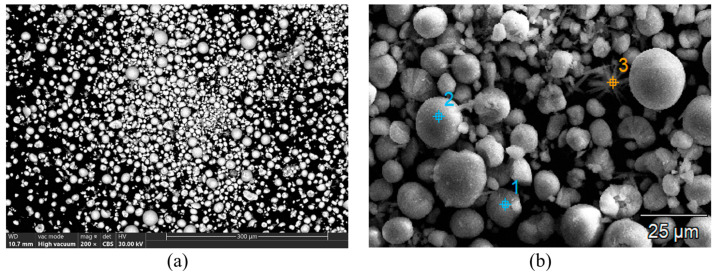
(a) The globular aspect of the particles obtained after ultrasonication of the cathode of used Ni-MH batteries; (b) the spots corresponding to the EDX analysis performed on globular particles obtained after ultrasonication of the cathode of used Ni-MH batteries.
